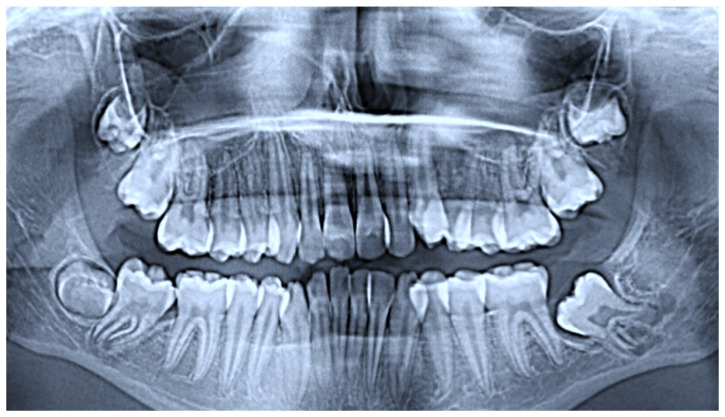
Panoramic radiograph at a 10-month follow-up.
